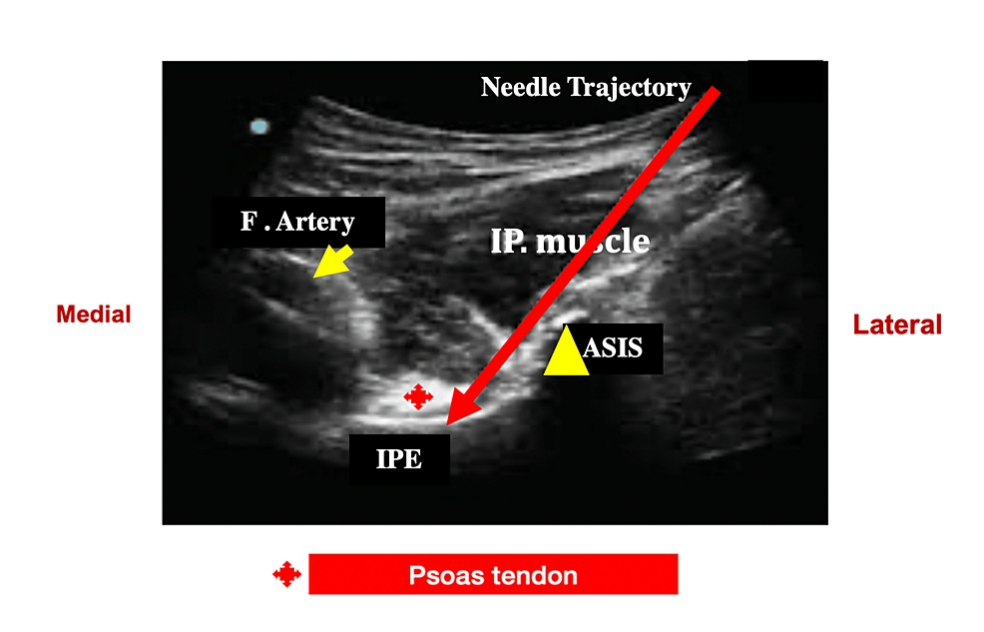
PENG block under ultrasound guidance PENG: pericapsular nerve group; ASIS: anterior superior iliac spine; IPE: iliopubic eminence.
